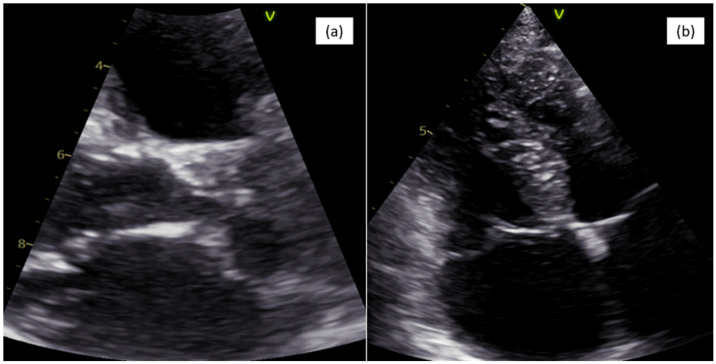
Echocardiography image: (a) parasternal long-axis view (PLAX) of unroofed coronary sinus and (b) right cavity enlargement.
